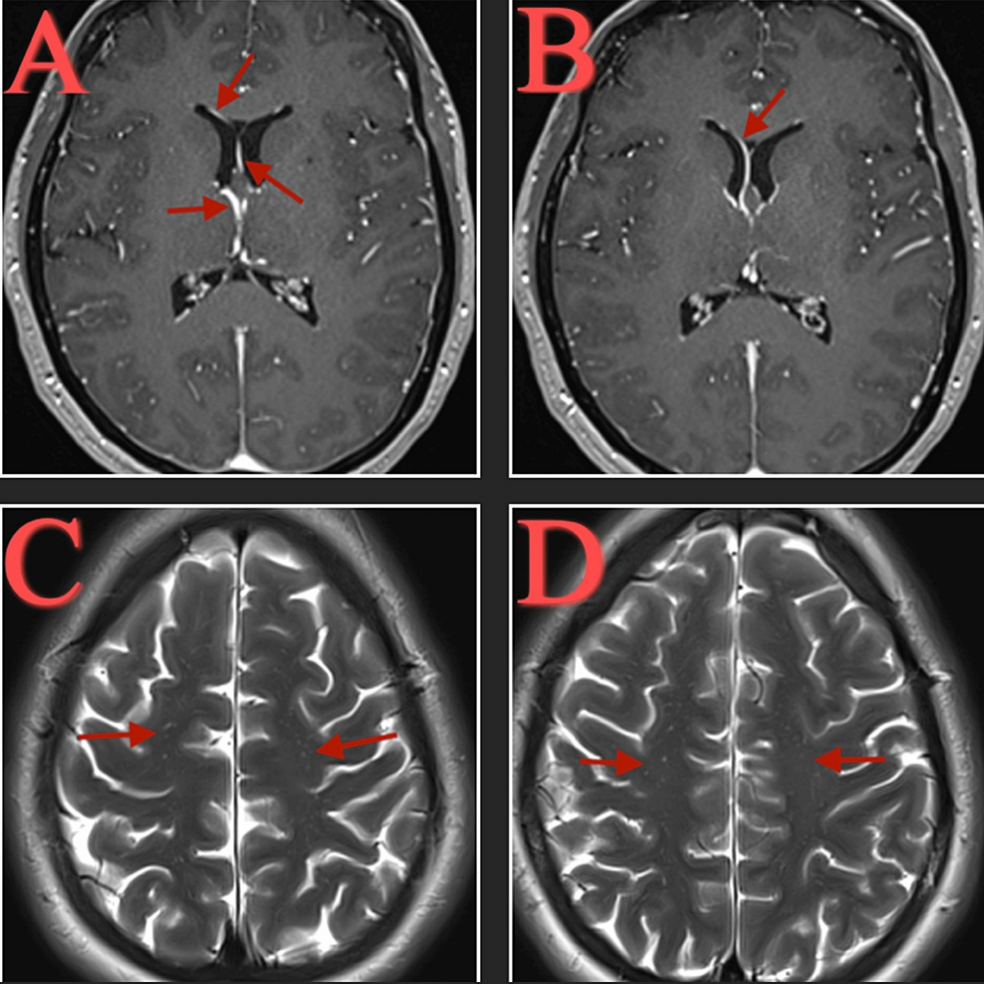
MRI brain with and without contrast. Persistent enhancement of ependymal linings signifying ventriculitis (A,B). Increased conspicuity of punctate foci of enhancement in the frontal and parietal corona radiata, corresponding to evolving tiny subacute infarcts (C,D).
